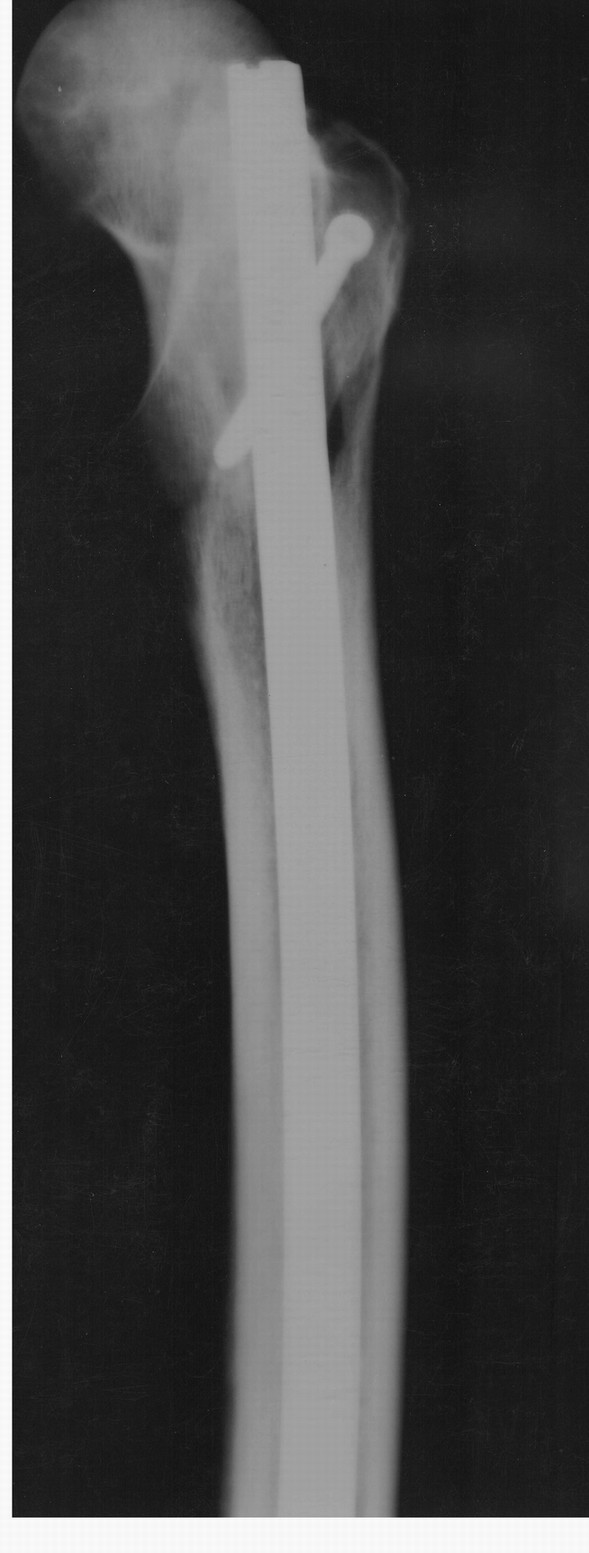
Lateral radiograph of the same femur. No evidence of fracture line can be documented.
